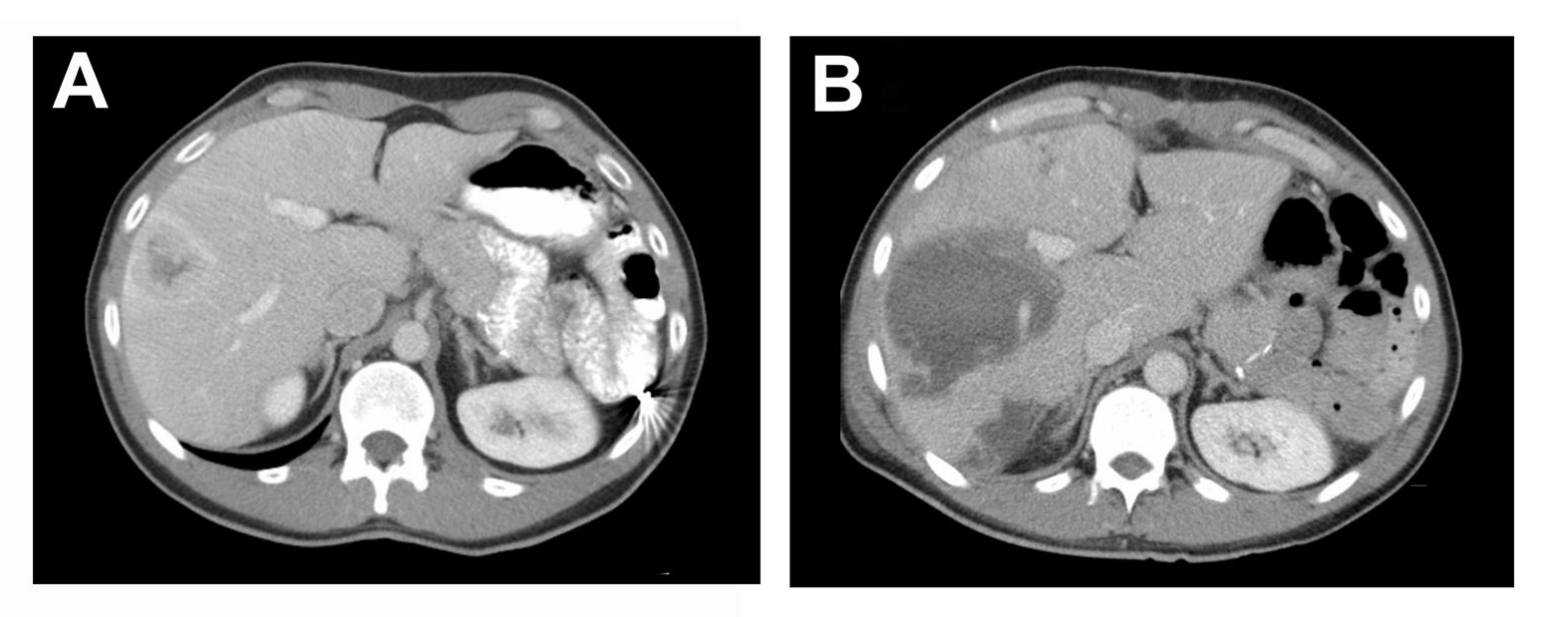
CT scan of the liver of a 34-year old man with metastases of a neuroendocrine tumour of the pancreas. Before LITT the CT scan shows a metastases of 4.7 cm in diameter in segment VII and a second metastases of 2.0 cm in diameter in segment VII subcapsular (not visible) of the liver (figure 2A). Control CT scan one week after LITT showing a coagulation lesion in segment VII of 9.0 cm in diameter and subcapsular in segment VII of 4.8 cm in diameter (figure 2B).
